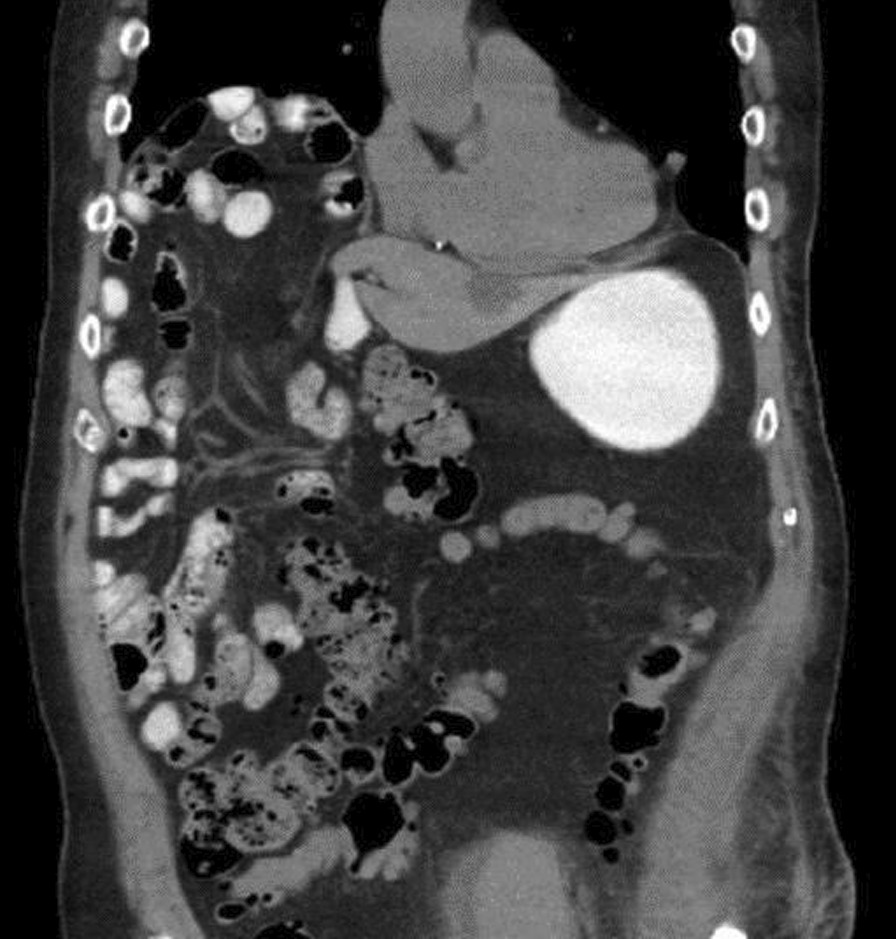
Location of proximal small bowel. CT of the abdomen and pelvis without intravenous contrast and with positive oral contrast. The entire small bowel is abnormally located in the right hemiabdomen and the colon predominantly to the left of the small bowel
